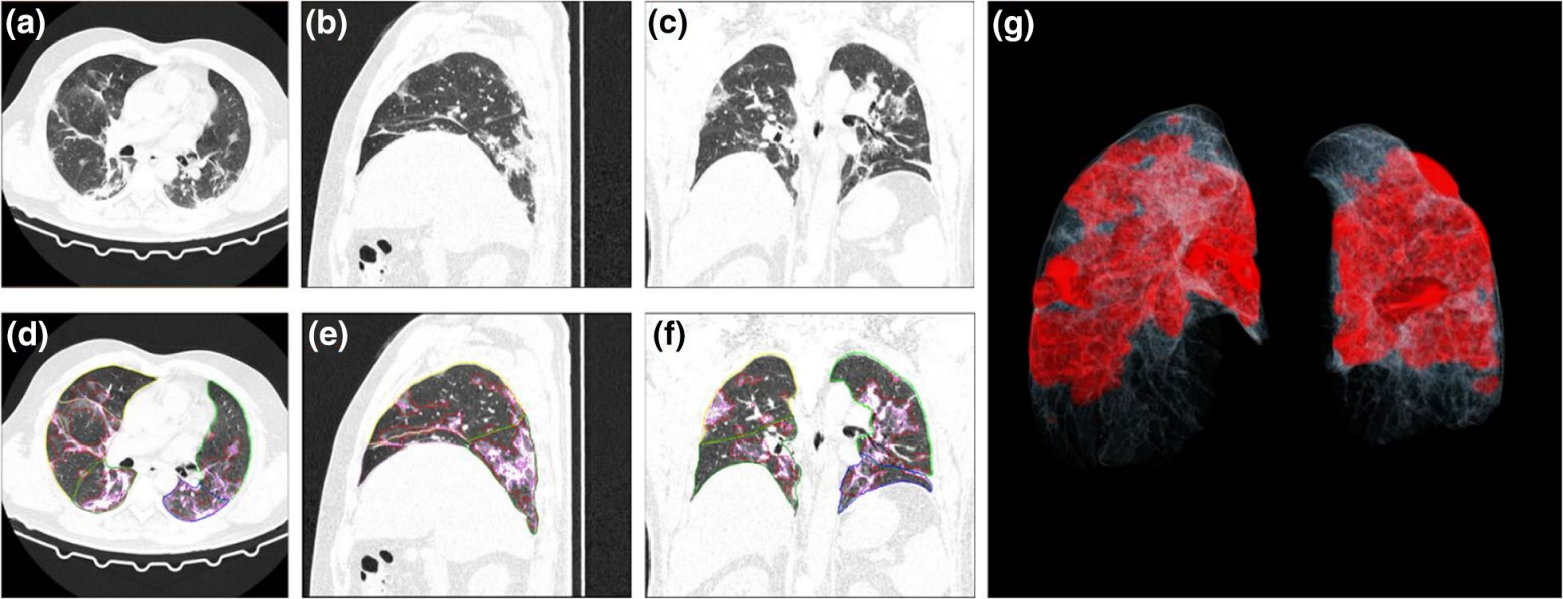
Anonymized HRCT images in axial (a), sagittal (b) and coronal (c) planes. Automated segmentation of lungs, lobes and abnormalities in same slices (d, e, f). 3D representation of lungs with abnormalities (g)
